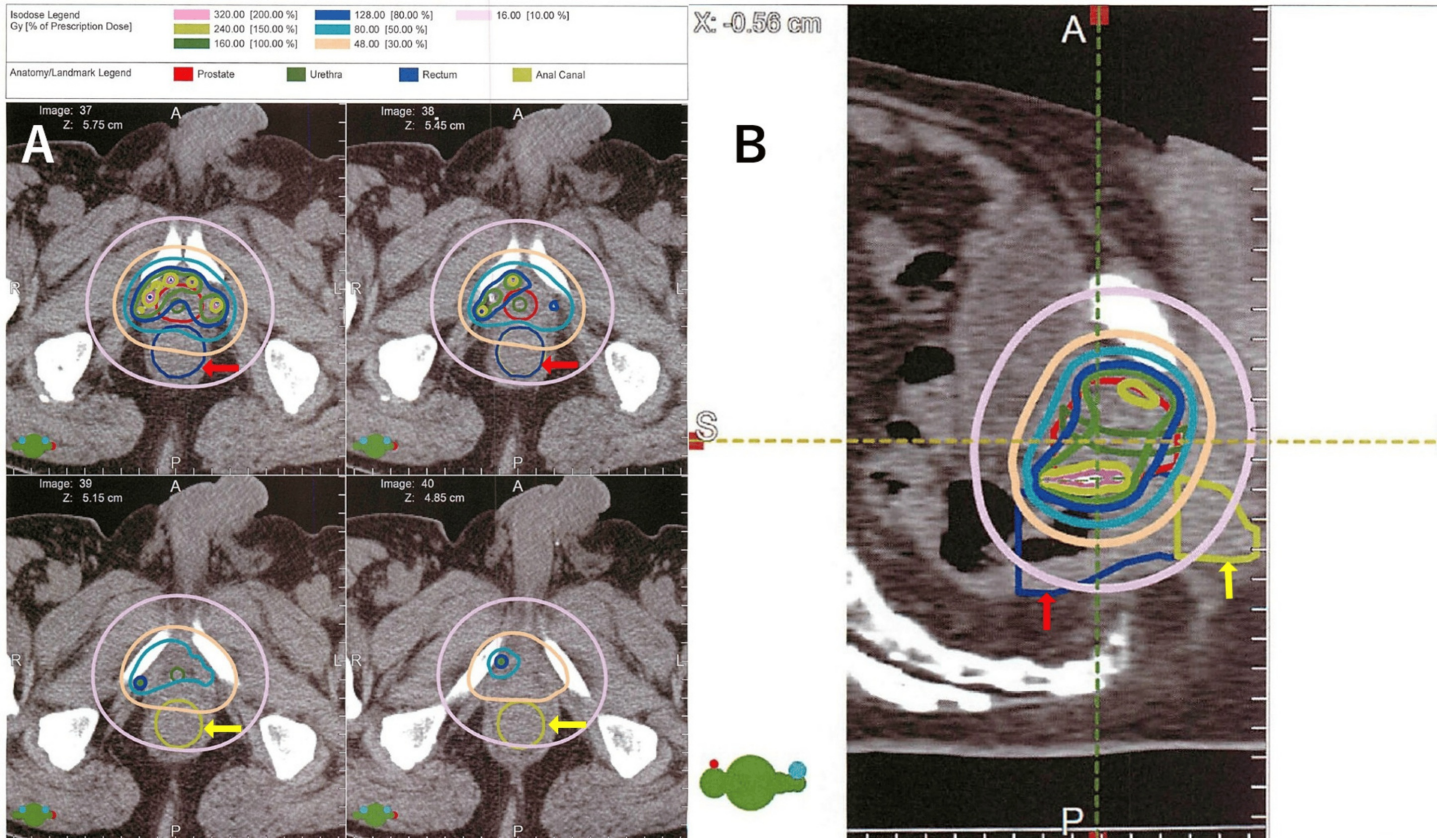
Post-implant dosimetry of Case 1 (A) Axial post-implant CT images showing the contoured rectum (red arrows) and anal canal (yellow arrows). (B) Sagittal reconstruction with isodose lines. The anal canal contour (yellow) demonstrates that although high-dose regions did not extend into the canal, low-to-moderate doses were delivered.
